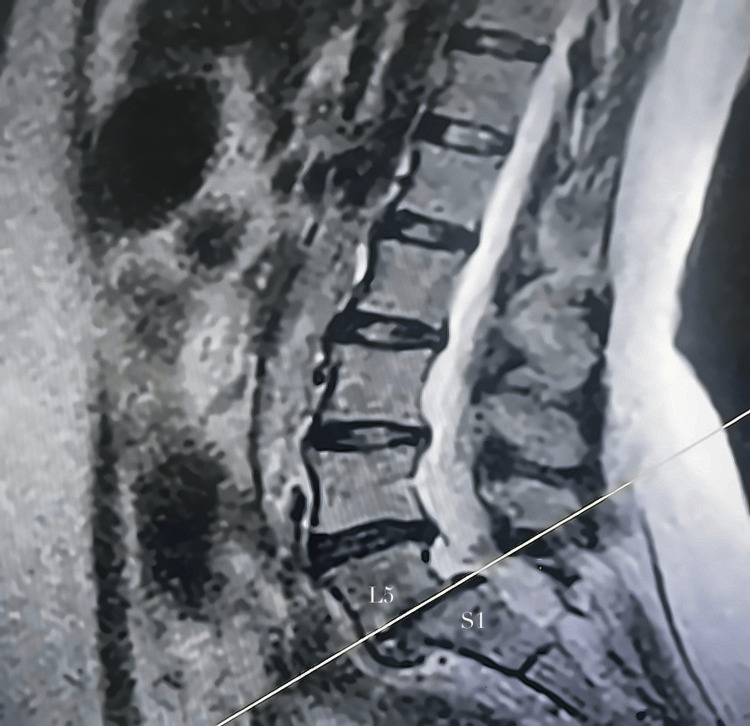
Lumbar spine MRI, sagittal view, demonstrating anterior displacement of L5 over S1 consistent with spondylolisthesis (white line)
